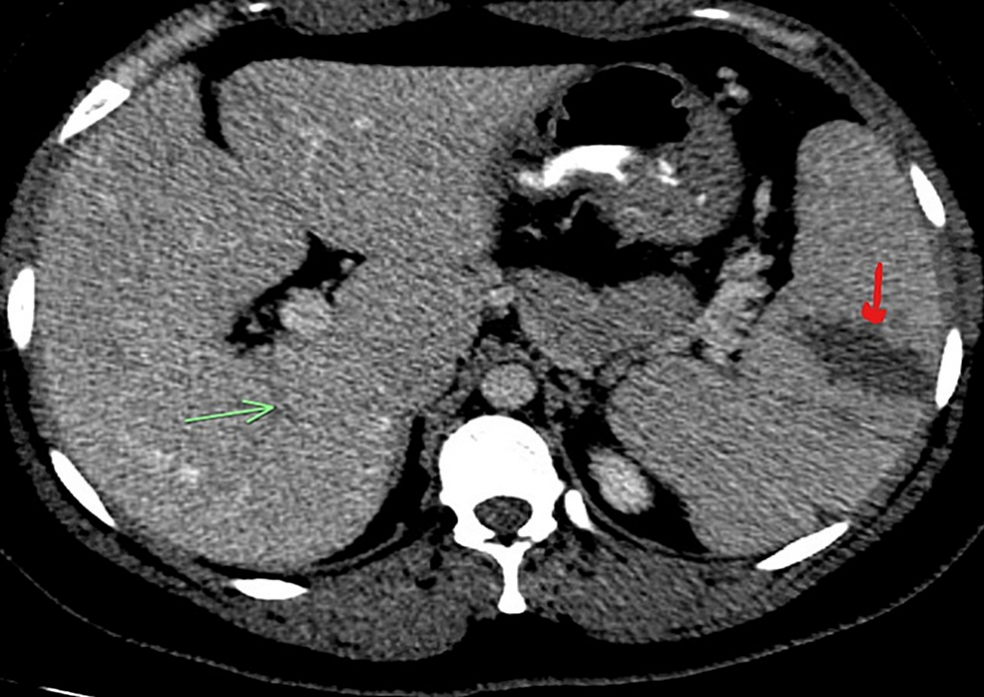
CT abdomen reveals non-opacification of the right portal vein (green arrow). On the right, splenic infarct within the parenchyma is notable (red arrow).
